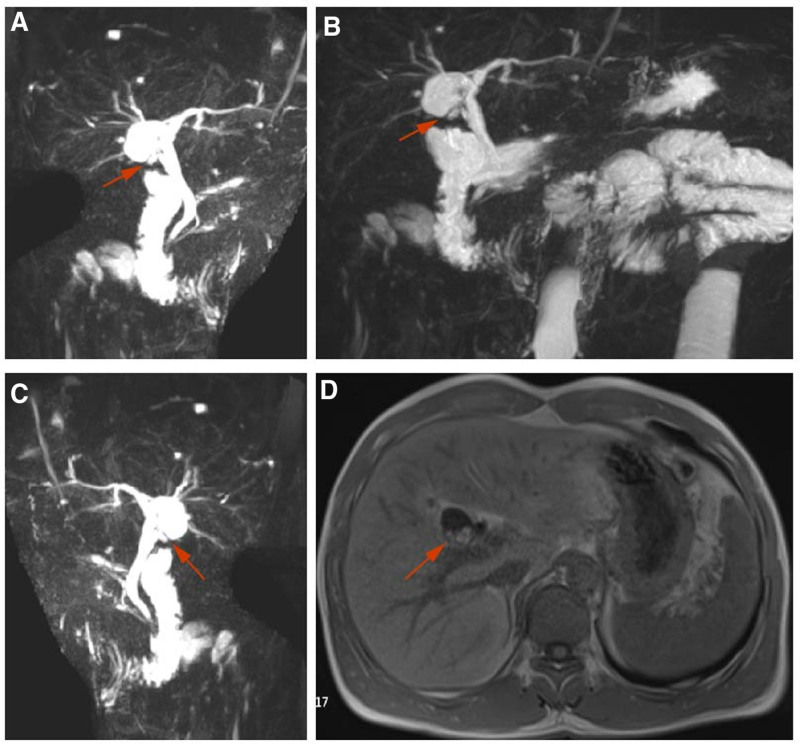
The patient’s MRCP. (A–C) Repeated gallbladder and residual cervical duct of gallbladder can be seen by MRCP radiography. (D) Repeated gallbladder stones can be seen on MRI. MRCP = magnetic resonance cholangiopancreatography.
